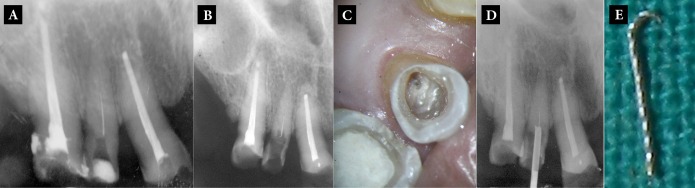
A) Periapical radiograph: Separated instrument is visible in middle 3rd of calcified root canal in maxillary right lateral incisor; B and C) Making a channel around the separated instrument to keep the broken instrument in the center of the tube of Masserann Kit; D and E) Engaging tube of Masserann Kit with the separated instrument and removal of the fragment from root canal
